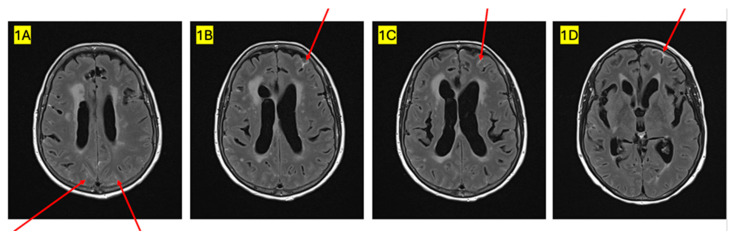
MRI FLAIR image demonstrating leptomeningeal enhancement within the parietal lobes bilaterally (1A), and multiple areas of leptomeningeal enhancement within the left frontal lobe (1B–1D).
